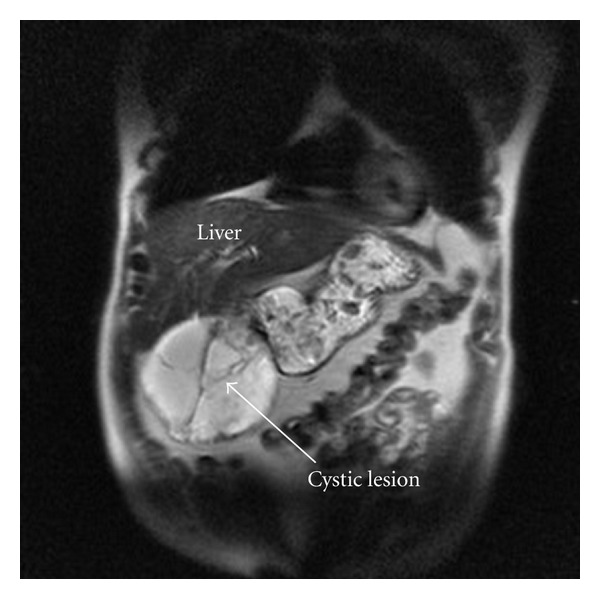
On MRI imaging markedly high intensity, compatible with cystic nature (arrow), was revealed in the tumor.
